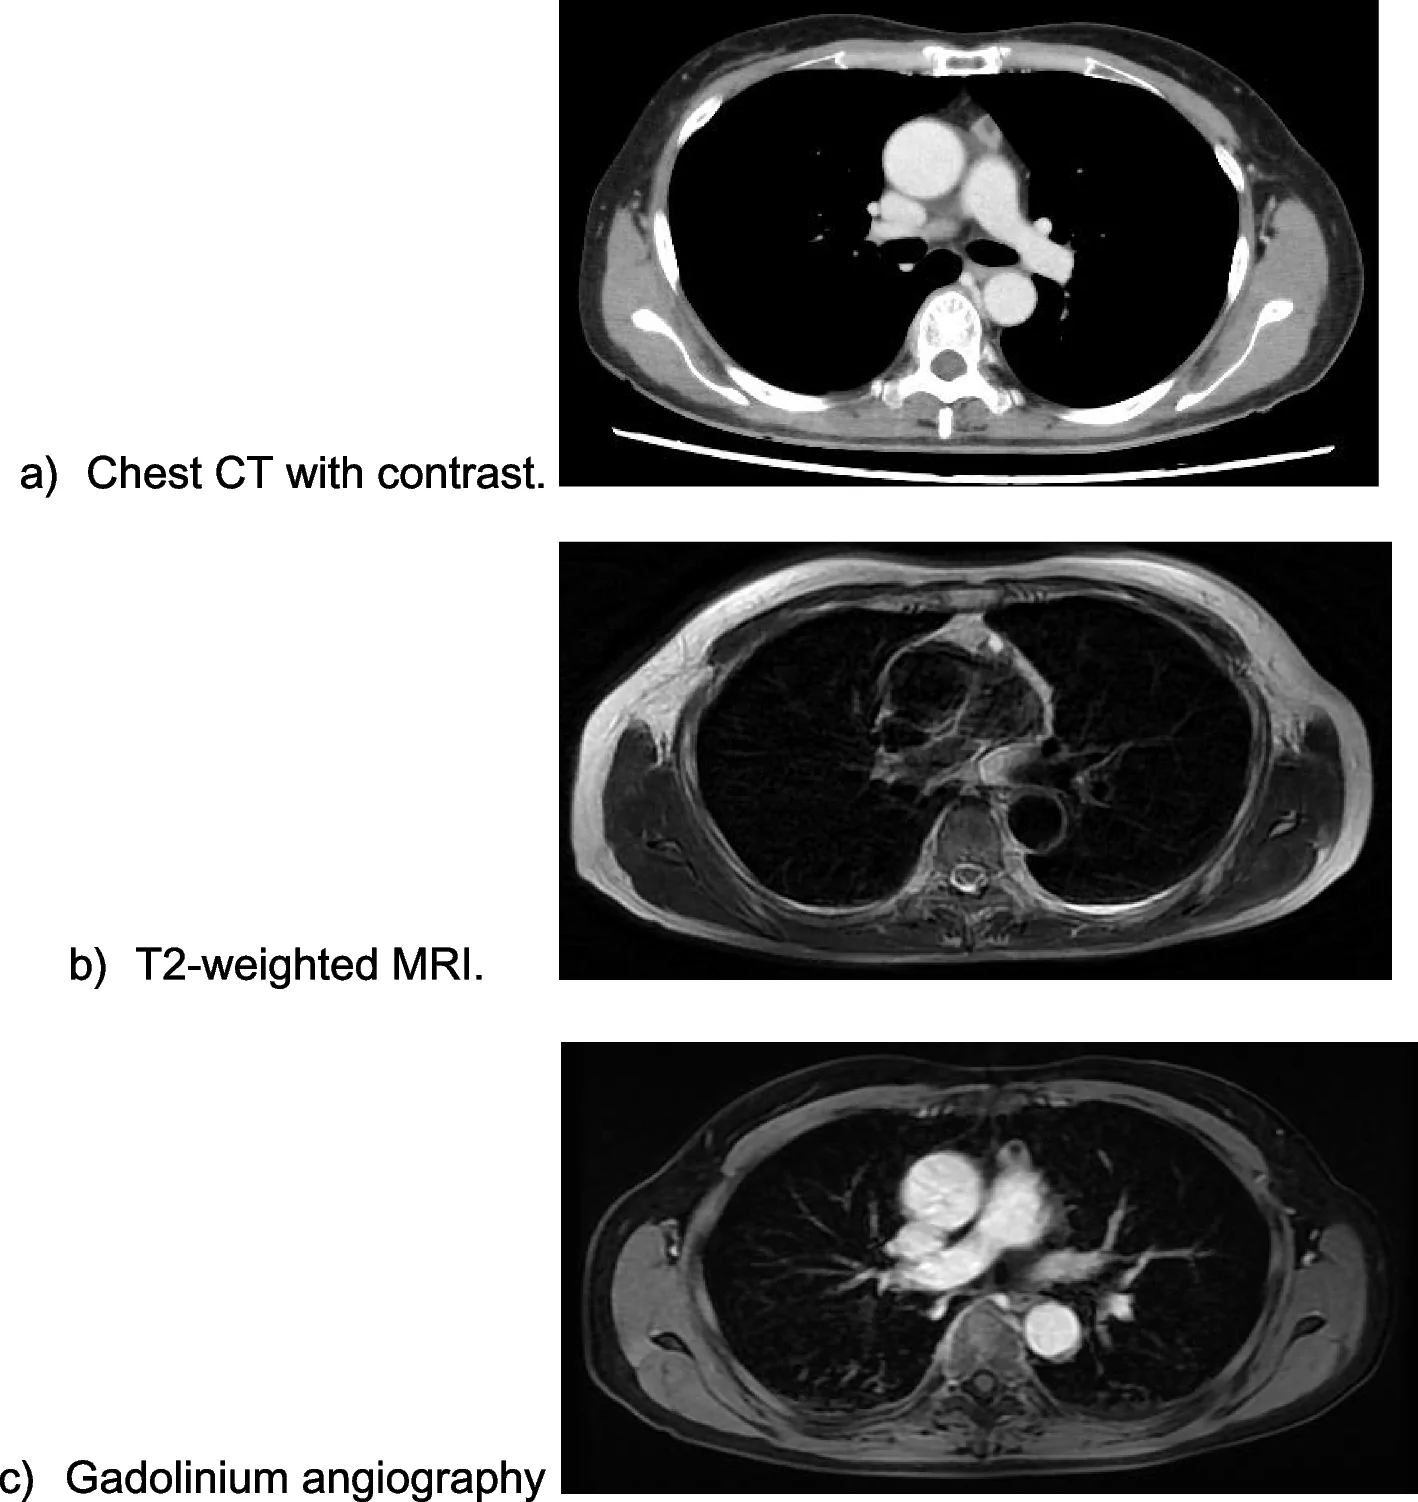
Chest computed tomography (CT) and magnetic resonance imaging (MRI) findings. An anterior mediastinal tumor measuring 13 mm in maximal diameter demonstrates partial contrast enhancement on contrast-enhanced CT (a). On T2-weighted MRI, the tumor exhibits areas of high signal intensity (b) with mild peripheral enhancement on gadolinium-enhanced images (c)
